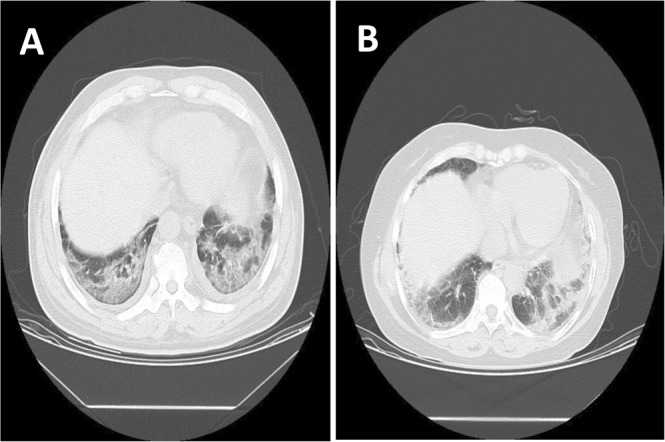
A paraquat poisoning patient (A) and a pneumonia patient (B) who were underwent CT scan.
